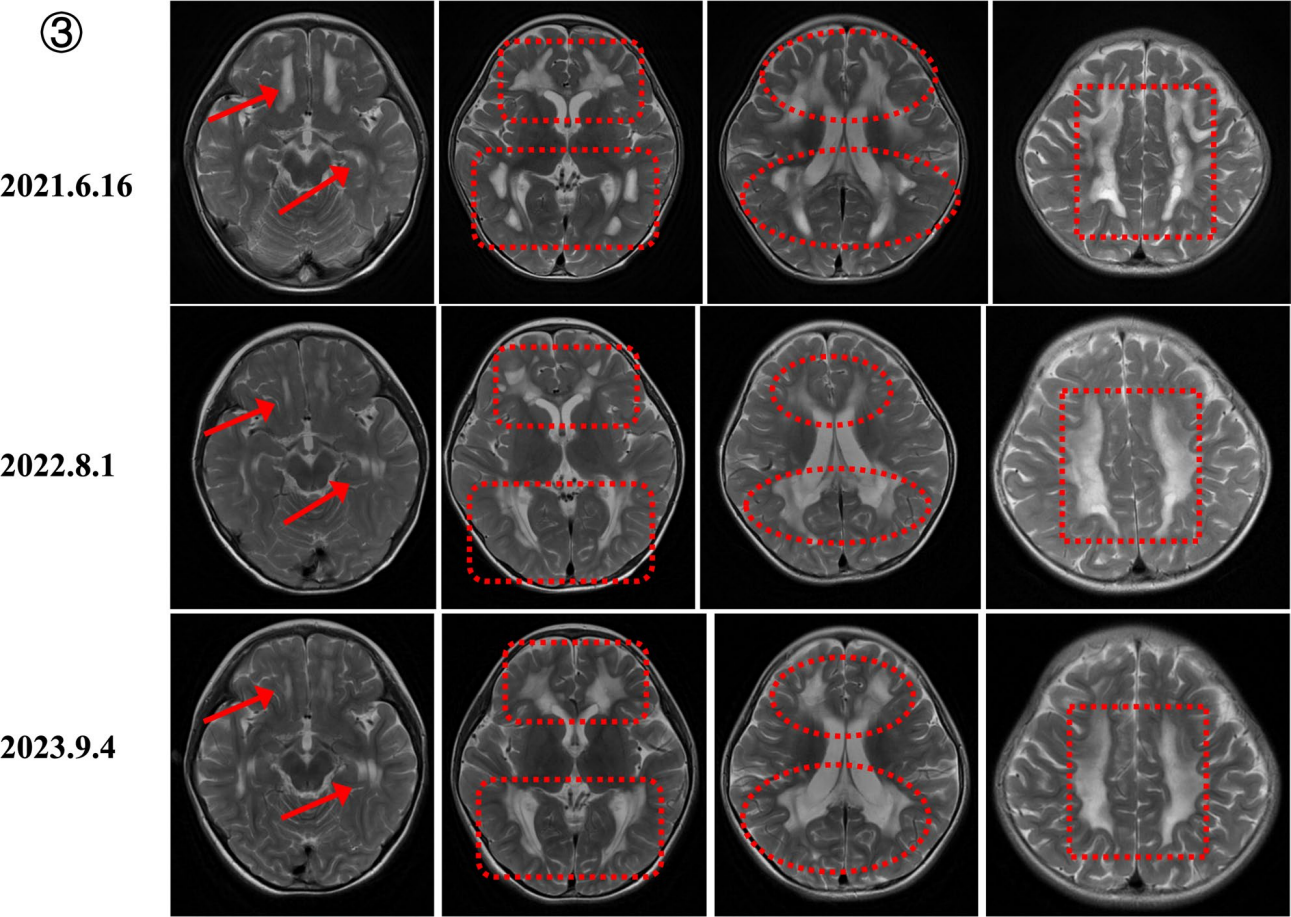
MRI evolution in NDUFS1-related complex I deficiency (2021–2023). (A) Initial cortical metabolic injury with cystic changes (2021). (B) Development of symmetric leukoencephalopathy involving the corpus callosum (2022). (C) Progressive white matter involvement (2023), reflecting a neurodegenerative trajectory refractory to mitochondrial therapy
